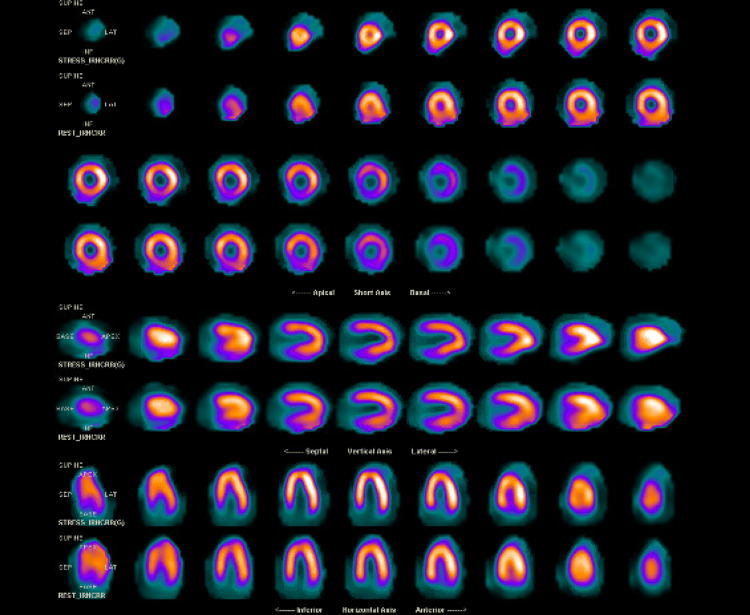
SPECT myocardial perfusion with no evidence of ischemia or scar SPECT: single-photon emission computed tomography
